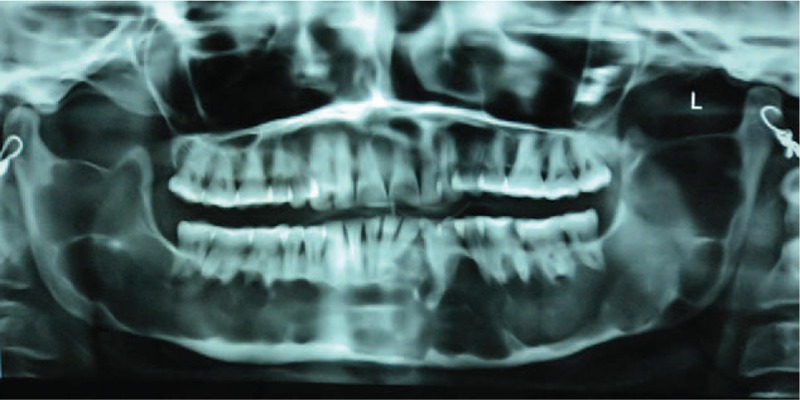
Preoperative radiograph showing an extensive radiolucent lesion spanning across the whole length of the mandible from one condyle to the other.
